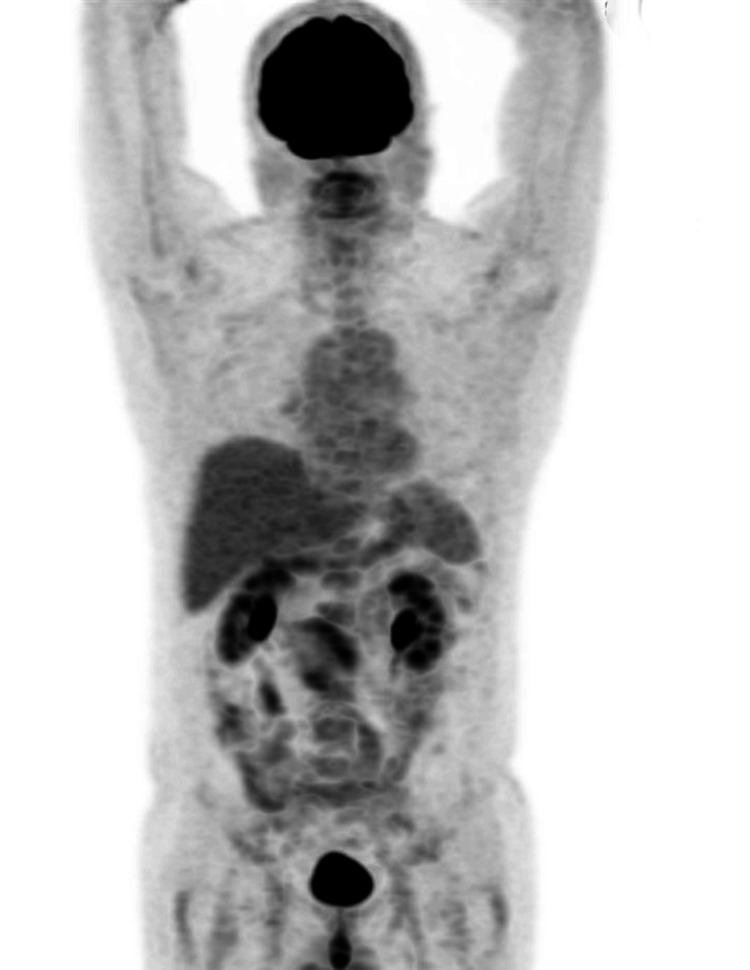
18-FDG PET scan performed in January 2024 was negative for oncological disease (complete response) 18-FDG: fludeoxyglucose F18; PET: positron emission tomography
